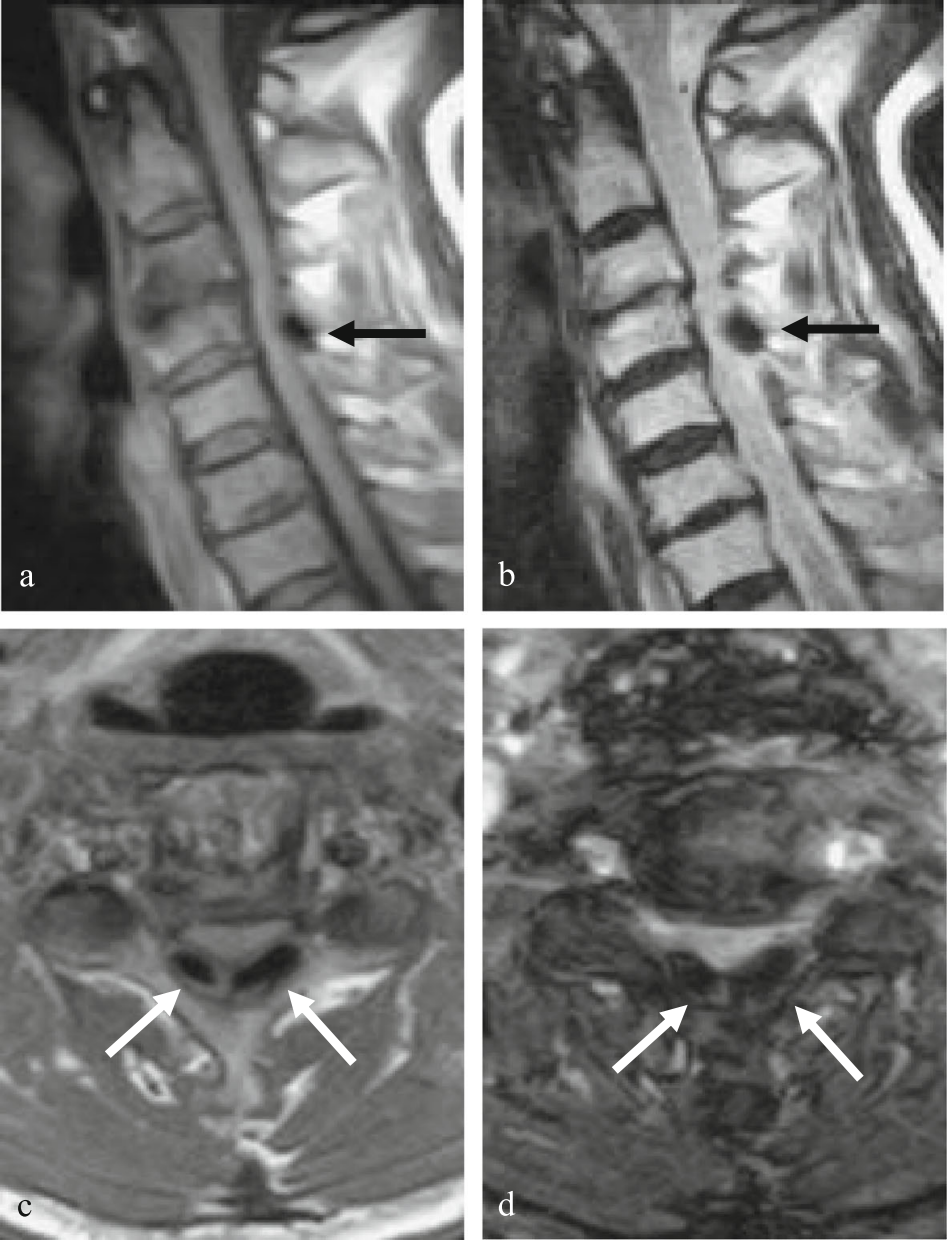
Sagittal (a and b) and axial (c and d) MRI demonstrated spinal cord compression by dark round lesions (black and white arrows) at C4 which appeared as a low signal mass on both T1 (a and c) and T2 (b and d) weighted images
